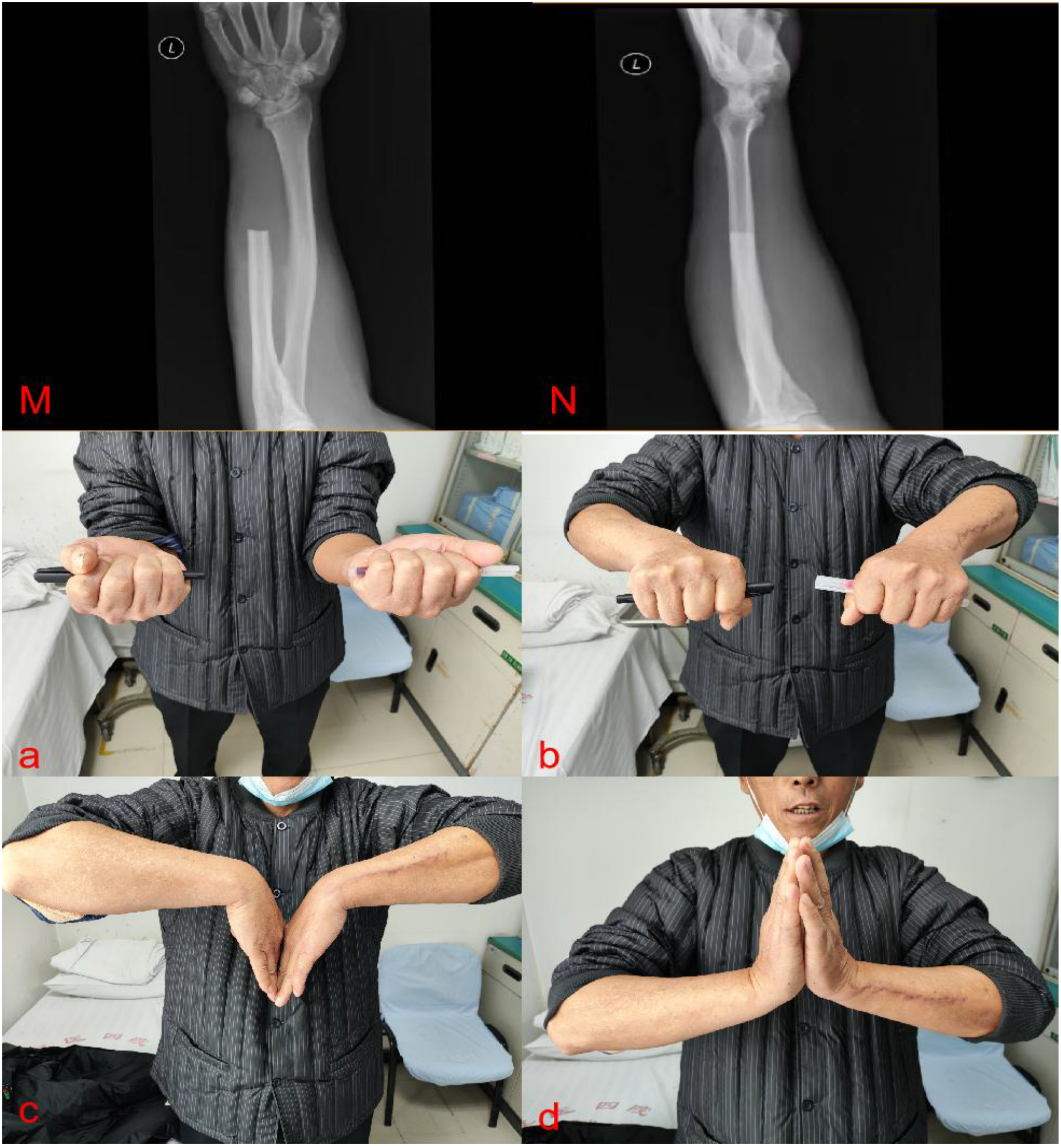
Postoperative radiographs of the ulna and radius. (M) positive position. (N) lateral view The patient could complete the full ROM, (a) pronation. (b) supination. (c) Palm flexion. (d), dorsiflexion.
